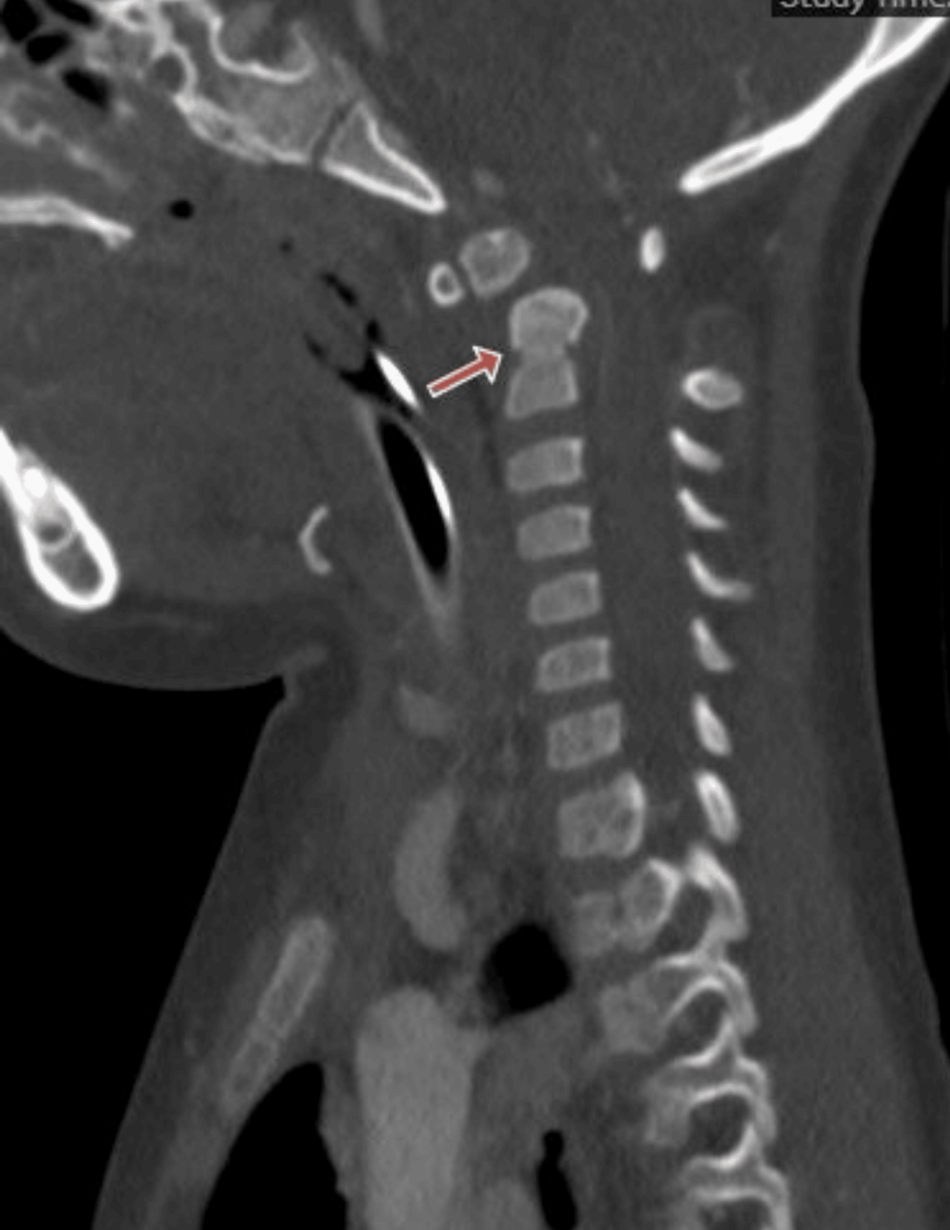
Sagittal sequence of the CT angiography showing os odontoideum with atlantoaxial subluxation (red arrow).
